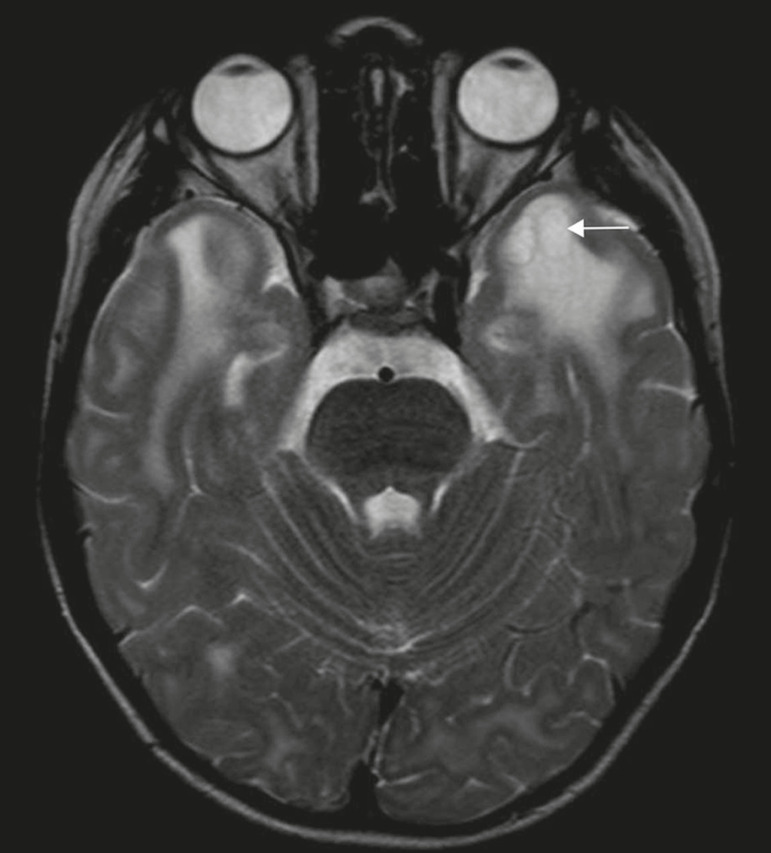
A 9-year-old male patient with macrocrania (since 5 months of age) and delayed neuropsychomotor development. Axial T2-weighted MRI sequence showing cysts in the left temporal pole (arrow). Note also the hyperintense signal in the subcortical and deep regions of the temporal lobes, as well as in the subcortical regions of the occipital lobe.
